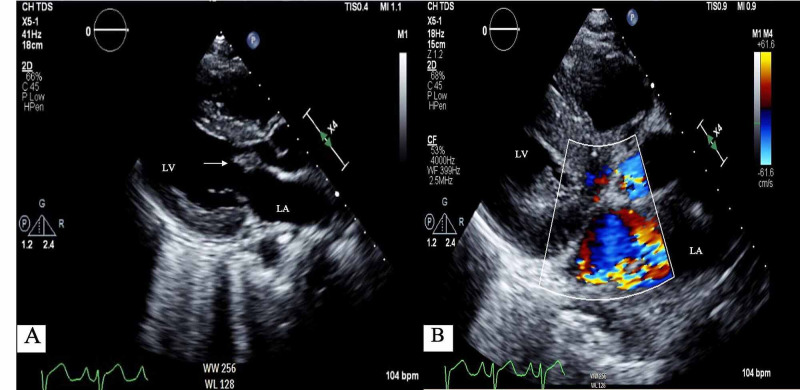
TTE. (A) Parasternal long axis view clearly shows vegetation of the anterior leaflet of mitral valve (arrow) with moderate-to-severe turbulent mitral regurgitation with eccentric jet to LA posterior wally. (B) LV end-diastolic dimension 4.1 cm, LV end-systolic dimension 2.7 cm, and LV ejection fraction (biplane) 68.3%. LA, left artial; LV, left ventricular; TTE, transesophageal echocardiography
